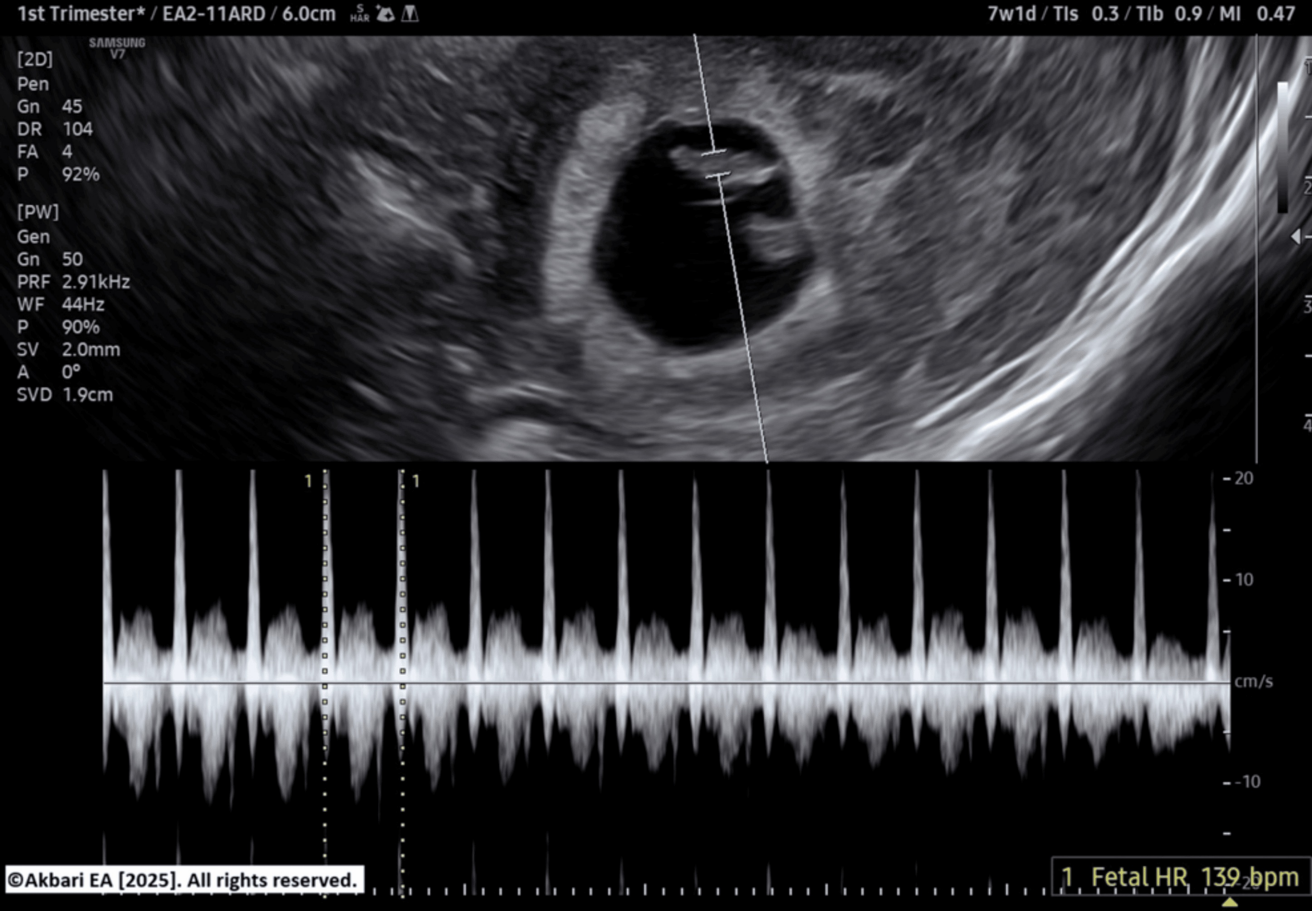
Transvaginal ultrasound image showing positive fetal heart at seven weeks of gestation Original image created by the author, ©Akbari EA 2025.
